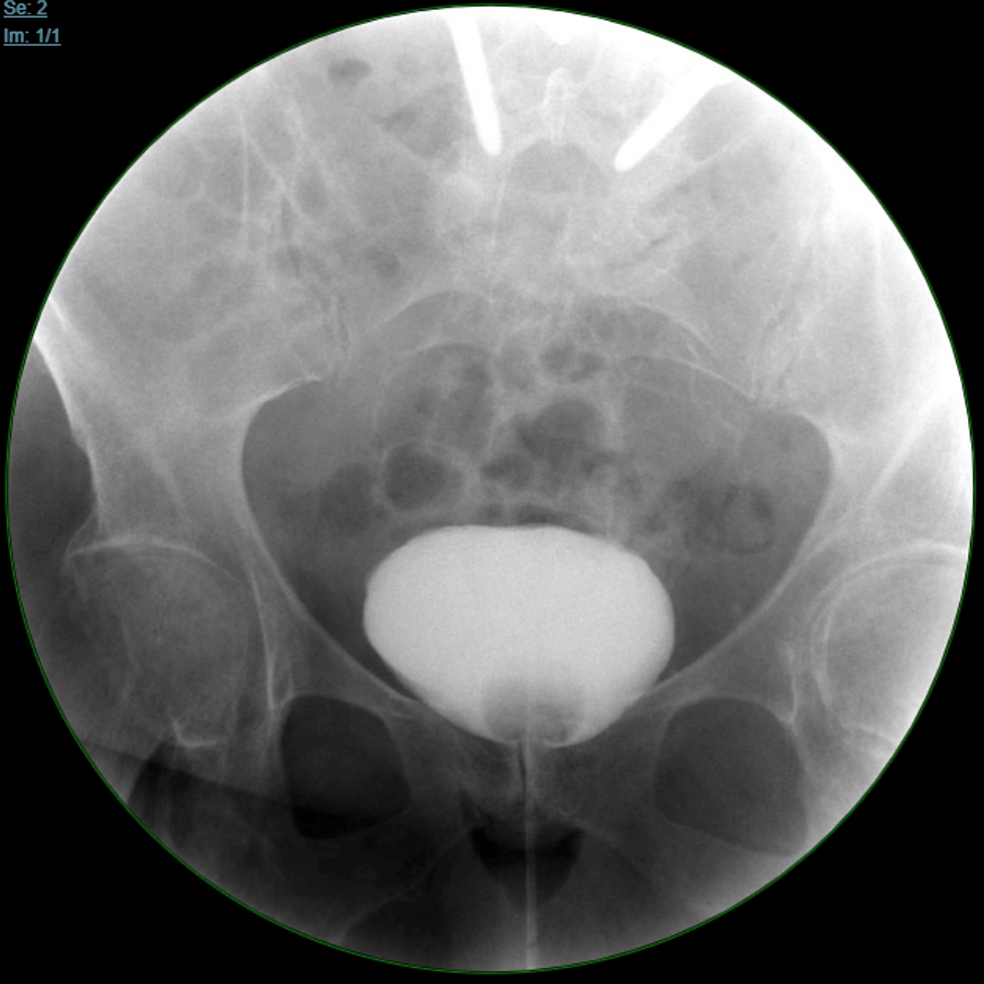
X-ray voiding urethrocystography (VCUG) revealed no reflux into the ureters.
